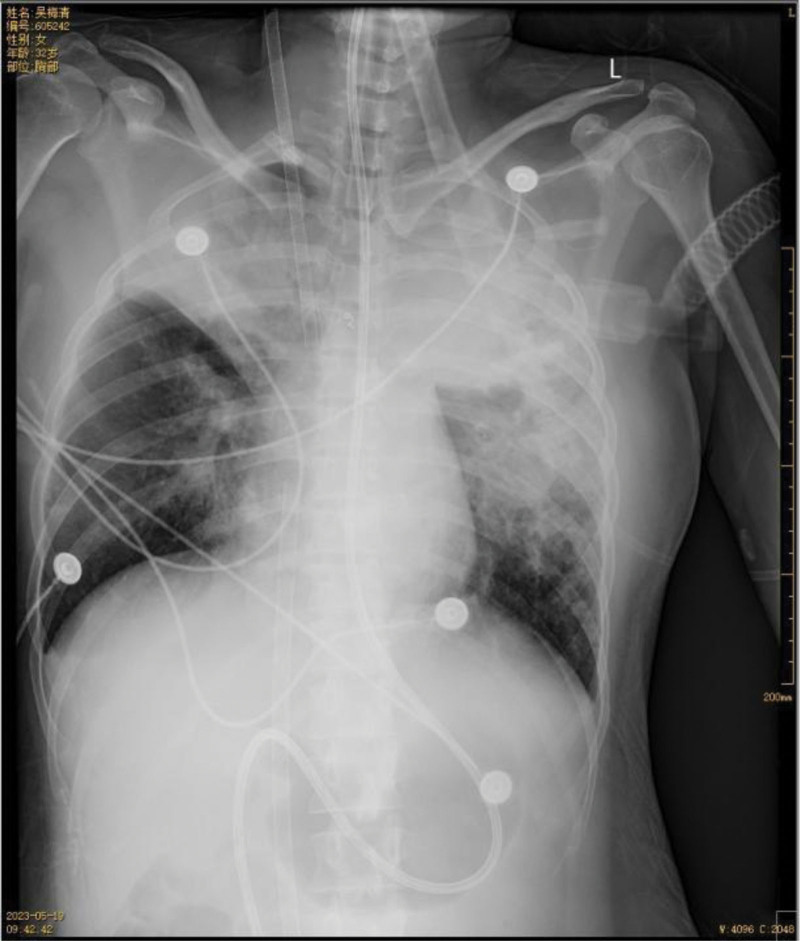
On May 19th, follow-up chest X-ray showed improvement in bilateral obstructive atelectasis compared to previous images.
